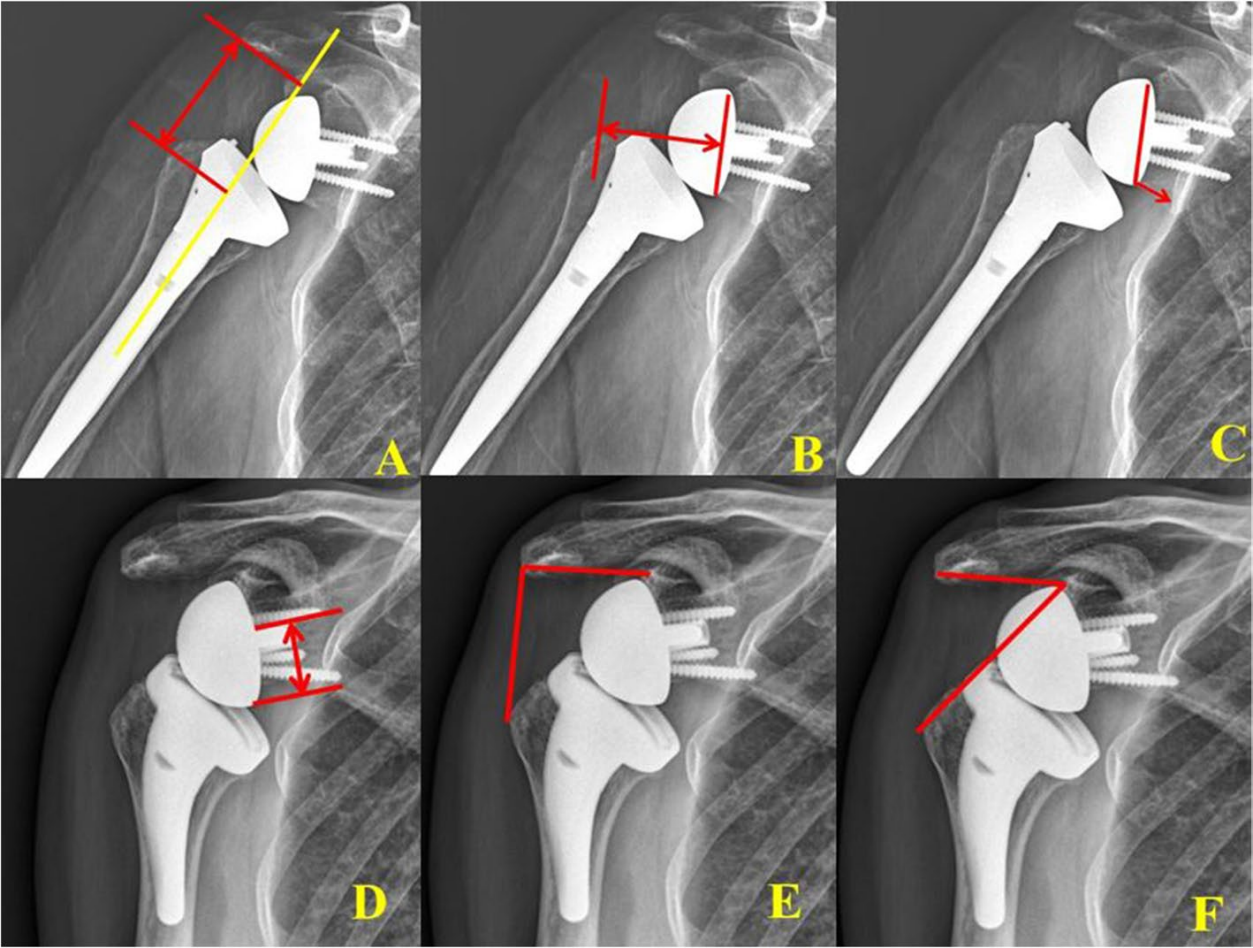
On the radiograph, we assessed several radiologic parameters and compared these between humeral and glenoid lateralization reverse total shoulder arthroplasty. A acromion-tuberosity (AT) distance, (B) glenoid-greater tuberosity (GT) distance*,* (C) prosthesis-scapular neck angle (PSNA) [20], (D) peg-glenoid rim distance (PGRD) [20], (E) lateralized shoulder angle, (F) distalization shoulder angle (DSA) [21]
